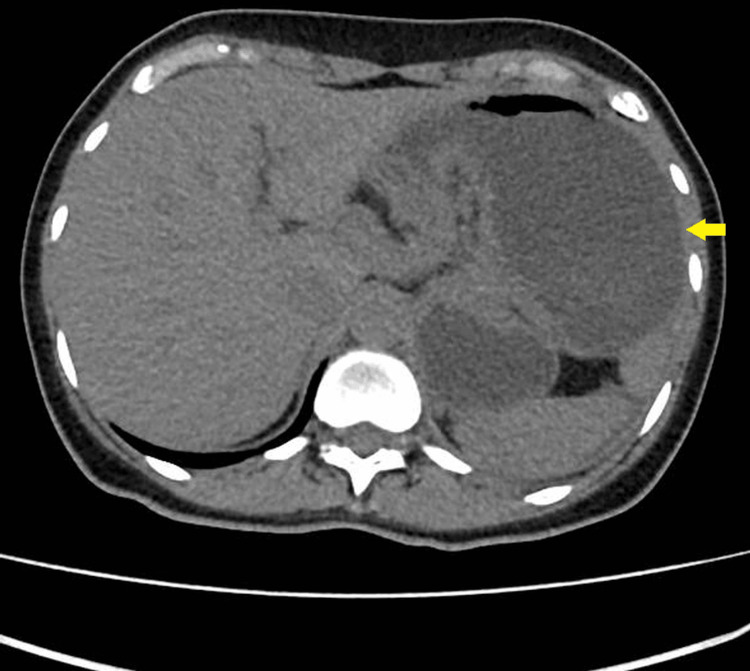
Axial view of the CT scan of the abdomen and pelvis demonstrating gastric volvulus CT: computed tomography
